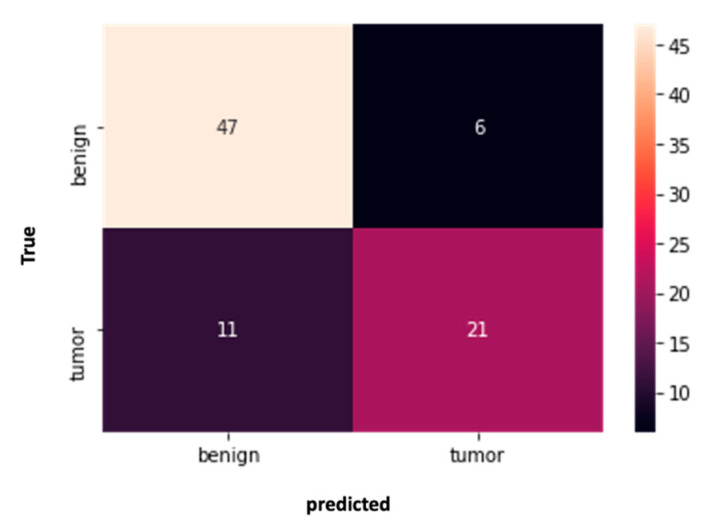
Confusion matrix of the performance of the network for classification of very early lesions/abnormalities in prior MRI scans to diagnosis scans of breast cancer.
